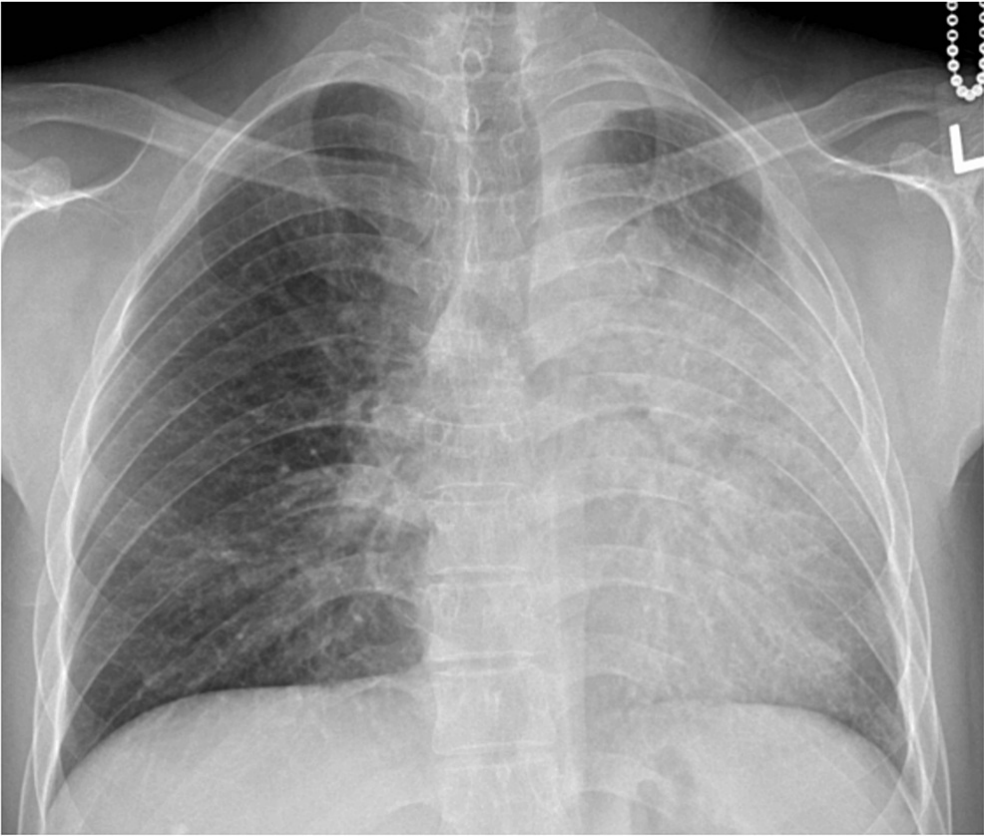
Chest X-ray showing LUL consolidation, possibly due to pneumonia or radiation (June 20, 2012; the last date of radiation was May 10) LUL: left upper lobe
